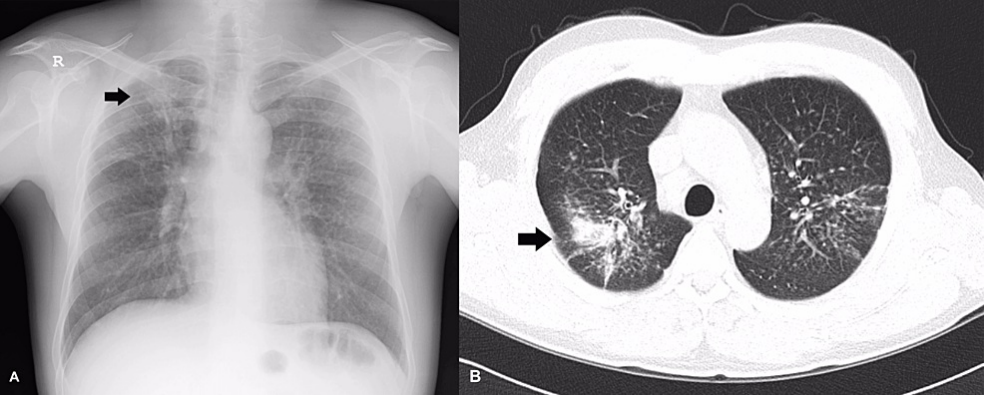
A. Chest radiograph shows multiple ill-defined small nodules in both lung fields, predominantly in the upper and middle zones with large opacities in the right upper lobe (black arrow). B. Axial thin-section computed tomography scan obtained multiple rounded centrilobular nodules in both lung fields with upper lobe dominance. There are conglomerated massive changes in the right upper lobe (black arrow) and coalescence of the small nodule in the left upper lobe.
